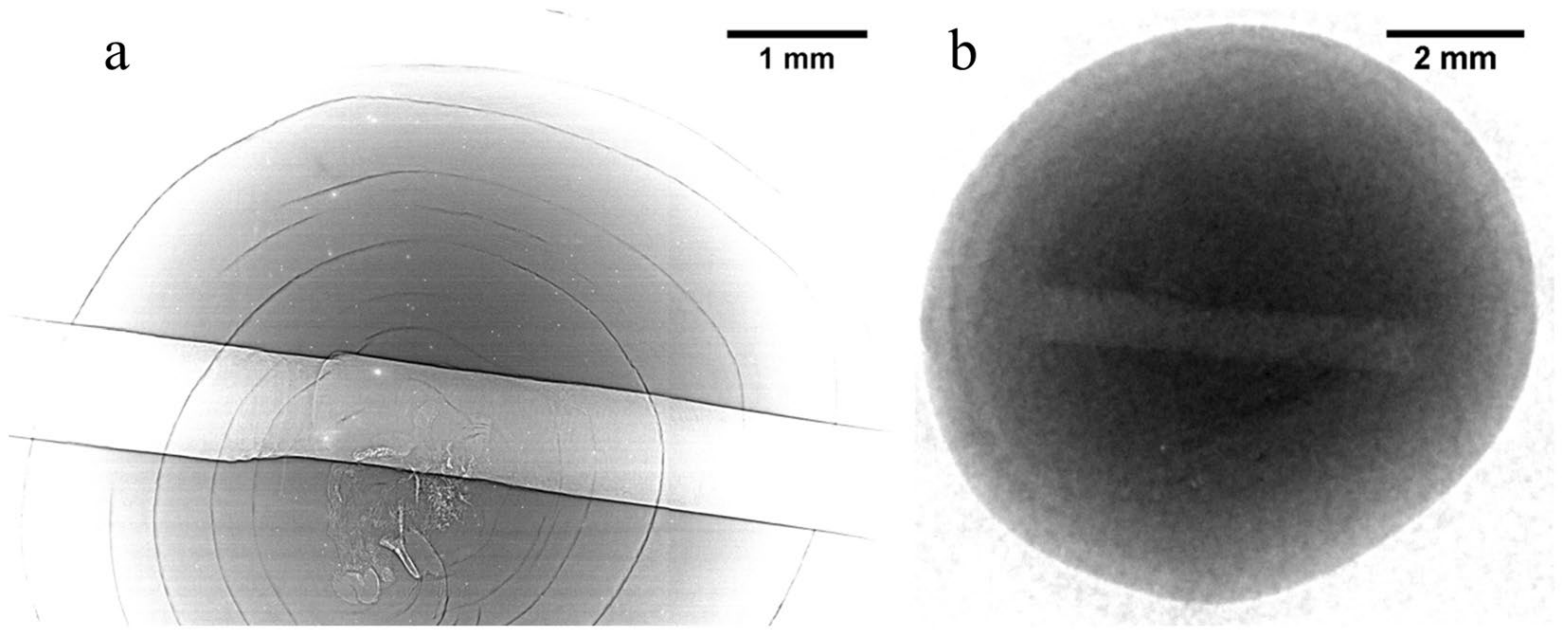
X-ray PC (a) and neutron radiographs (b) of the cultured freshwater pearl NP-21. In (a) the contrast is maximized in order to highlight the growth rings and inner voids of the pearl. The drill-hole is clearly visible in both images.
